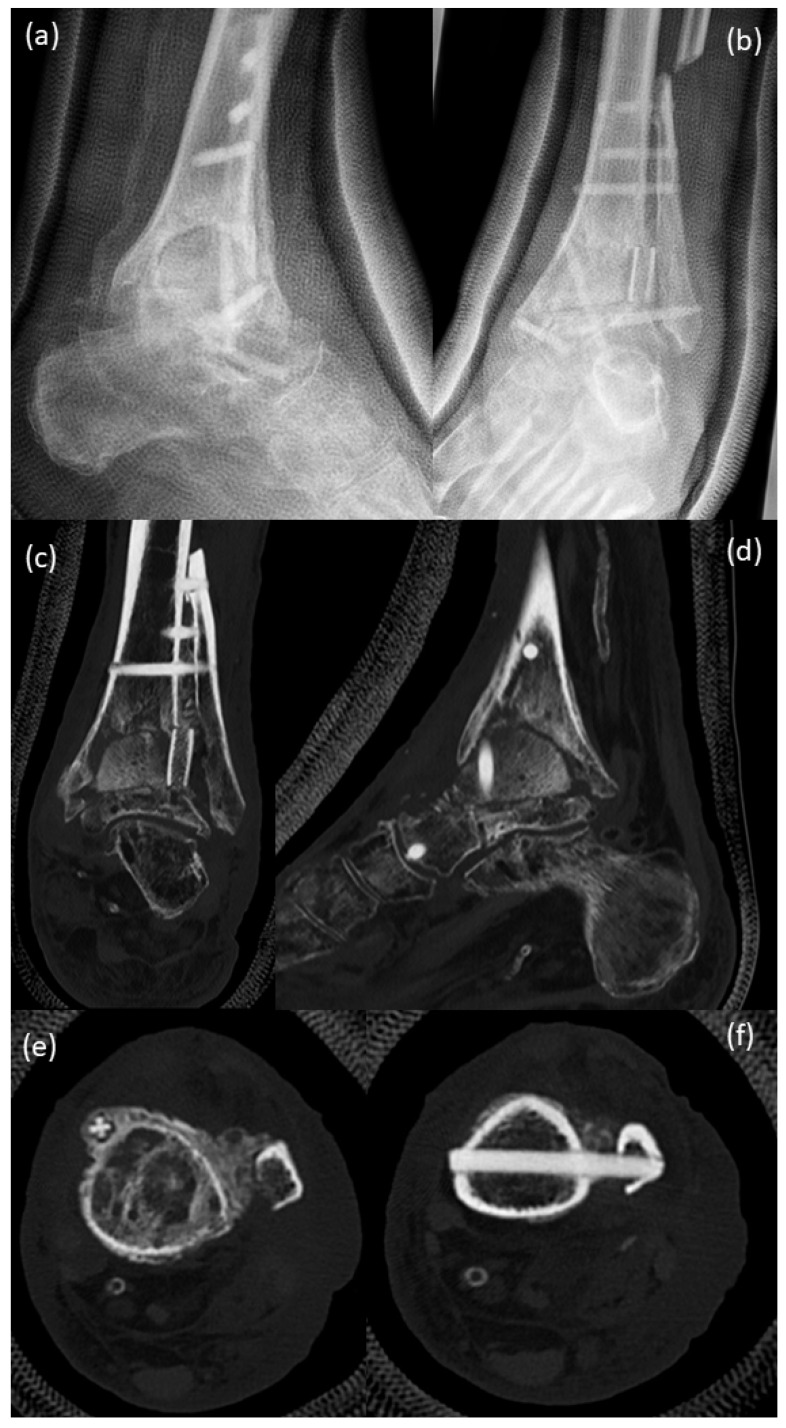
(a,b) X-ray; (c–f) CT scans 12 weeks after surgery with a clearly visible formation of callosal bone being seen around the screws with the beginning of bony consolidation.
